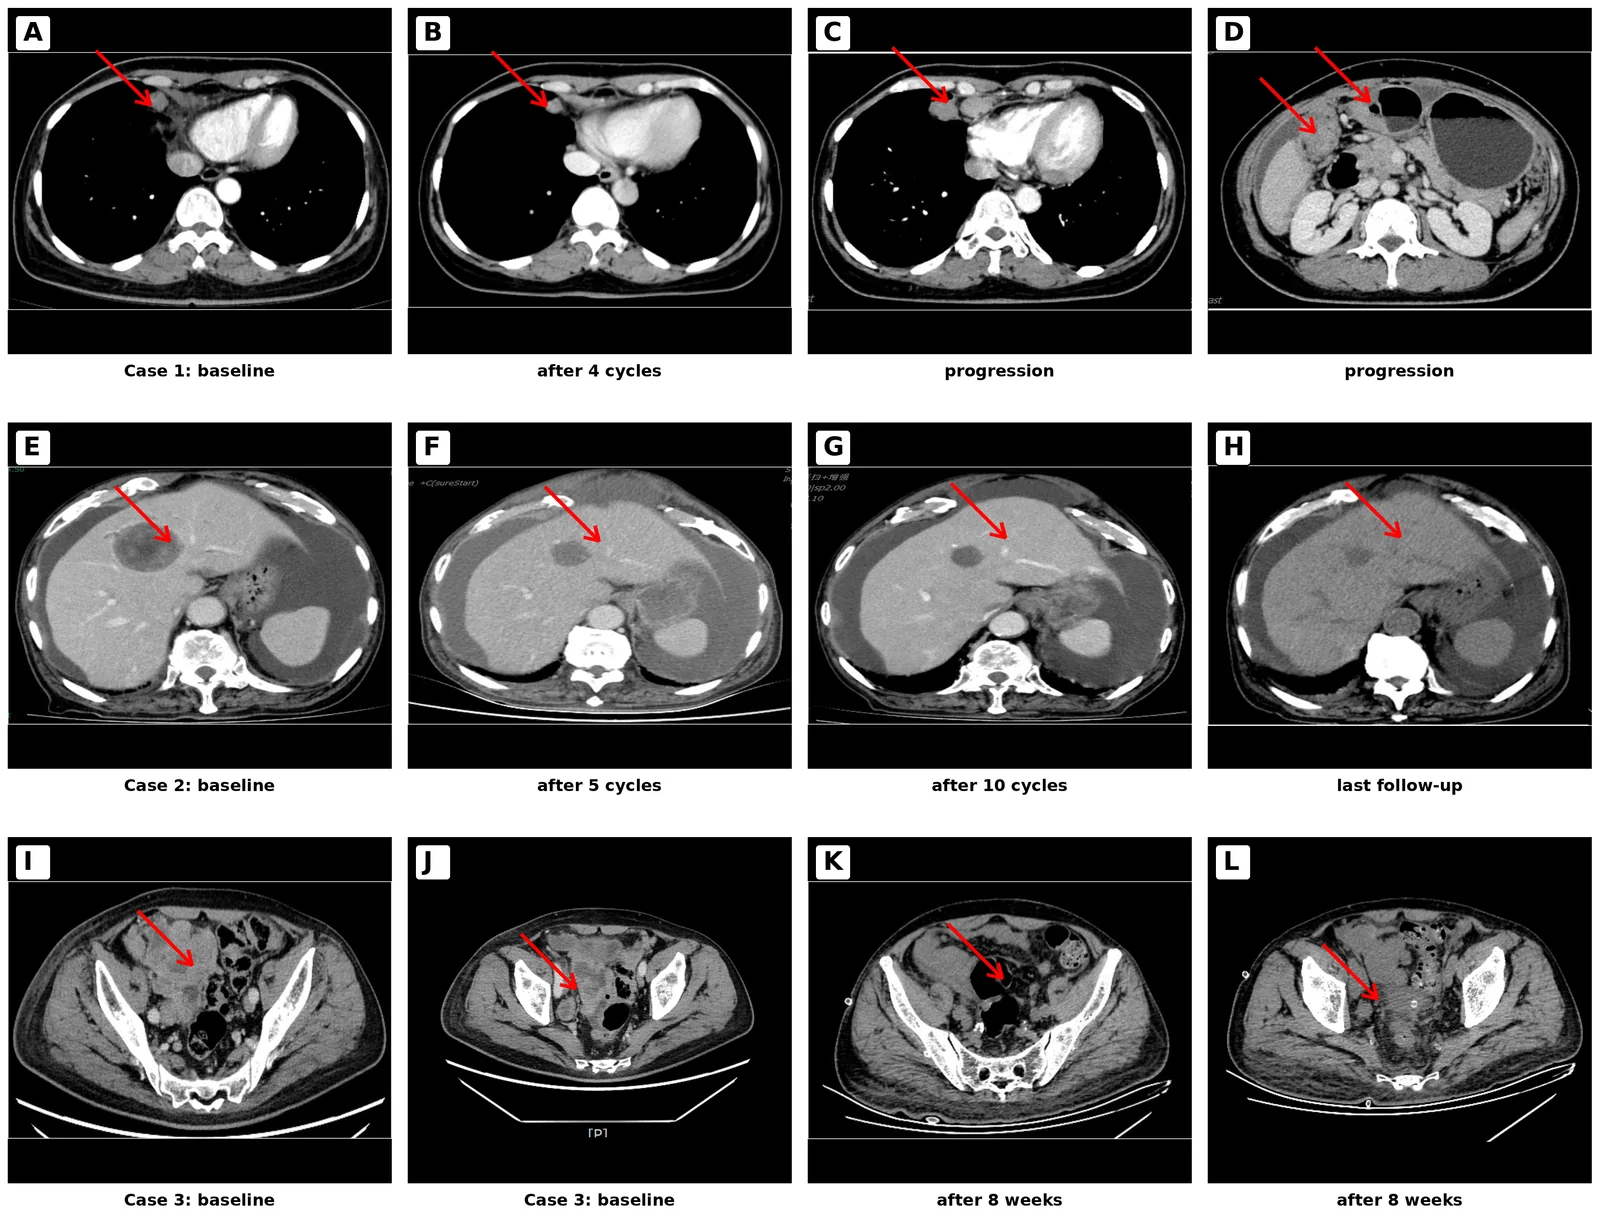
Serial CT findings before and after treatment in the three patients. (A–D) Case 1: cardiophrenic and peritoneal lesions at baseline, after four treatment cycles, and at progression. (E–H) Case 2: hepatic lesions at baseline, after five cycles, after 10 cycles, and at the most recent follow-up in February 2026. (I–L) Case 3: two representative abdominopelvic levels at baseline and after approximately 8 weeks of QL1706 treatment. Red arrows indicate representative lesions.
